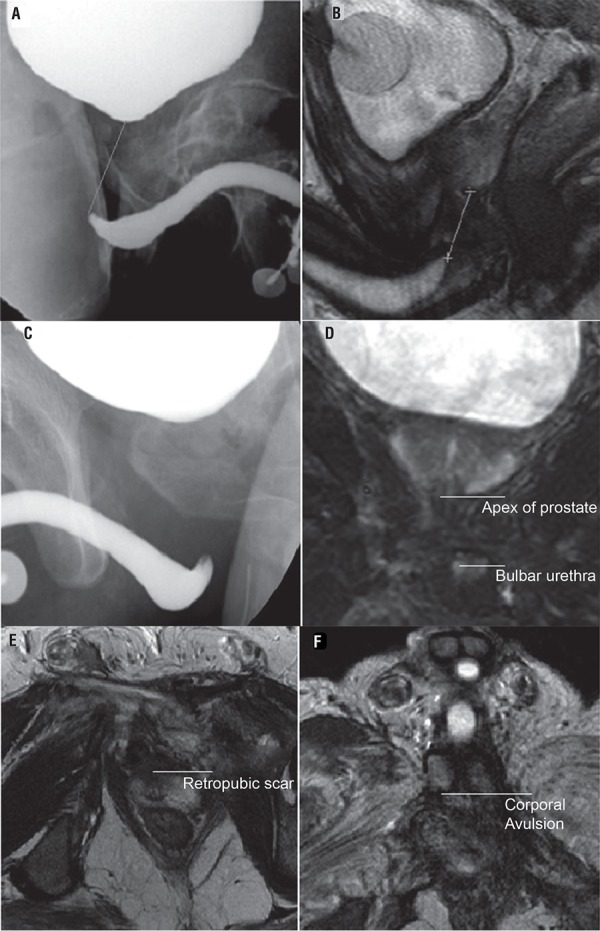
The figure shows urethral defect on MCU (A) and MRI (B); prostatic displacement on MCU (C) and MRI (D); retropubic scarring on MRI (E); and corporal avulsion on MRI (F).
